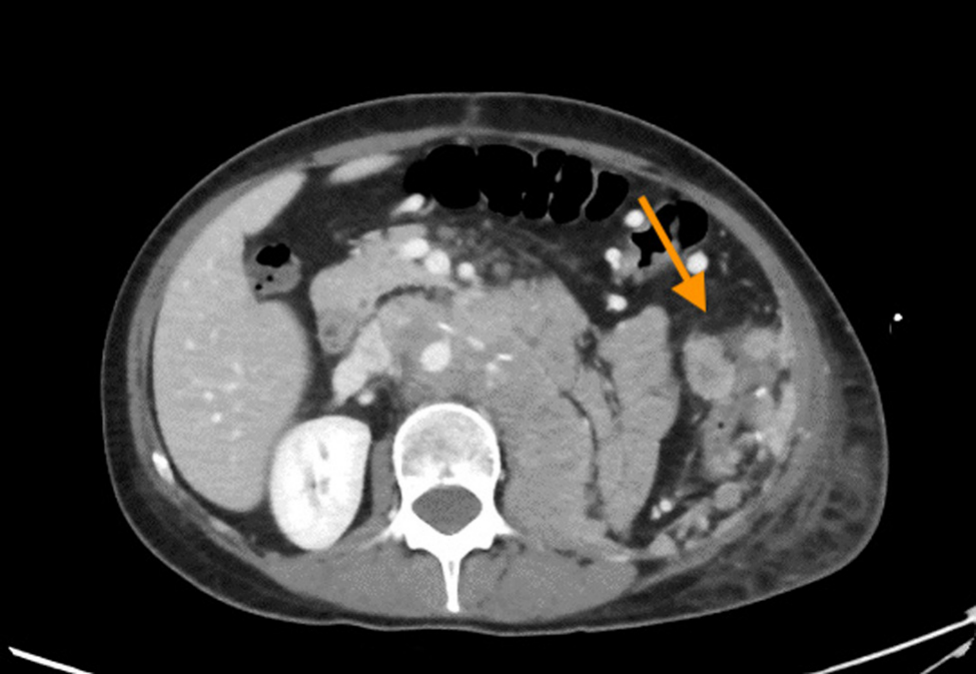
CT-scan, the imaging revealed a peritoneal carcinomatosis (arrow).
